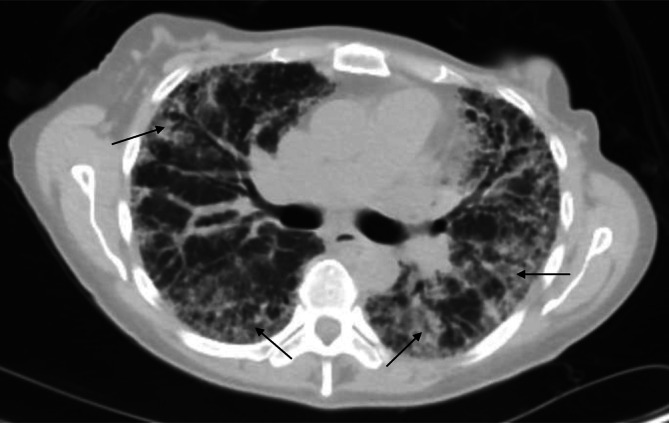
High resolution computed tomography of the chest showing end‐stage extensive pulmonary fibrosis. Trans axial image demonstrates areas of interstitial fibrosis with predominant involvement of periphery and lung bases. Arrow indicates areas of fibrotic regions.
